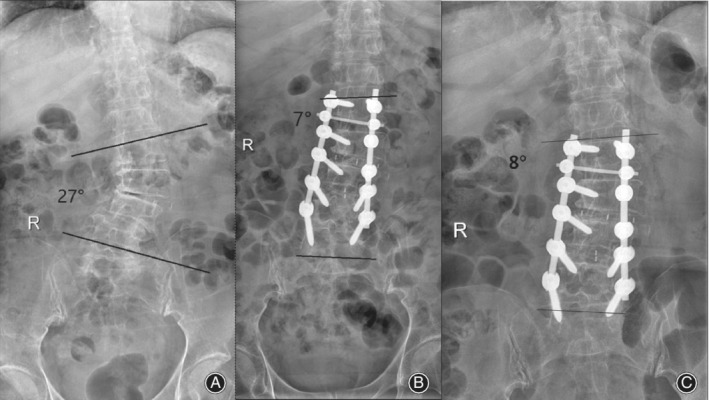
A 68‐year‐old woman underwent oblique lumbar interbody fusion (OLIF). The anteroposterior (A) X‐ray radiographs show scoliosis deformity and the Cobb angle is 27°. The X‐ray radiographs (B) and (C) at 1 week and 5 months postoperatively, respectively, show that the Cobb angle decreased 19° and the deformity was rectified remarkably.
